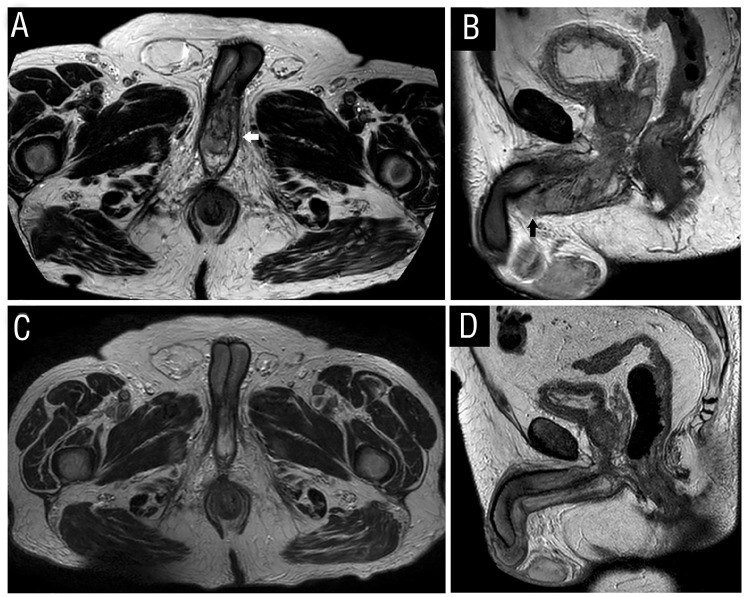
(A-D) – MRI of the urethral metastasis (T2WI). Axial (A) and sagittal (B) images show an area of marked T2 heterogeneity involving the bulb (white arrow in A) and proximal body of the penis (black arrow in B) with a gross cylindrical shape. This lesion has an estimated total extension of 10 cm. It is presumably centred in the urethra (penile, bulbous and membranous) with loss of the normal anatomic planes between the urethra and the surrounding corpus spongiosum. The described lesion corresponds to an area with heterogeneous contrast enhancement after gadolinium injection and restricted diffusion (not shown). Followup MRI of the pelvis after three therapeutic cycles with sunitinib revealed a reduction of the lesion's extension and less heterogeneity, with a better definition of the anatomical planes (C and D). There was no significant restriction to diffusion on DWI (not shown).
